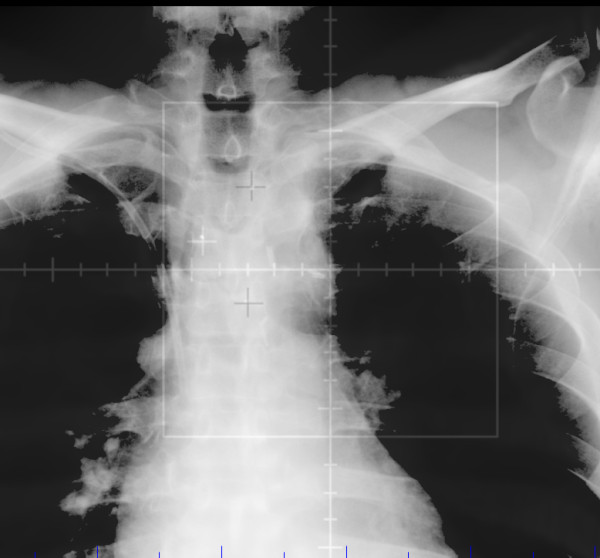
Matching of digital reconstructed radiograph to kilovoltage image using fiducial markers (anteroposterior view).
